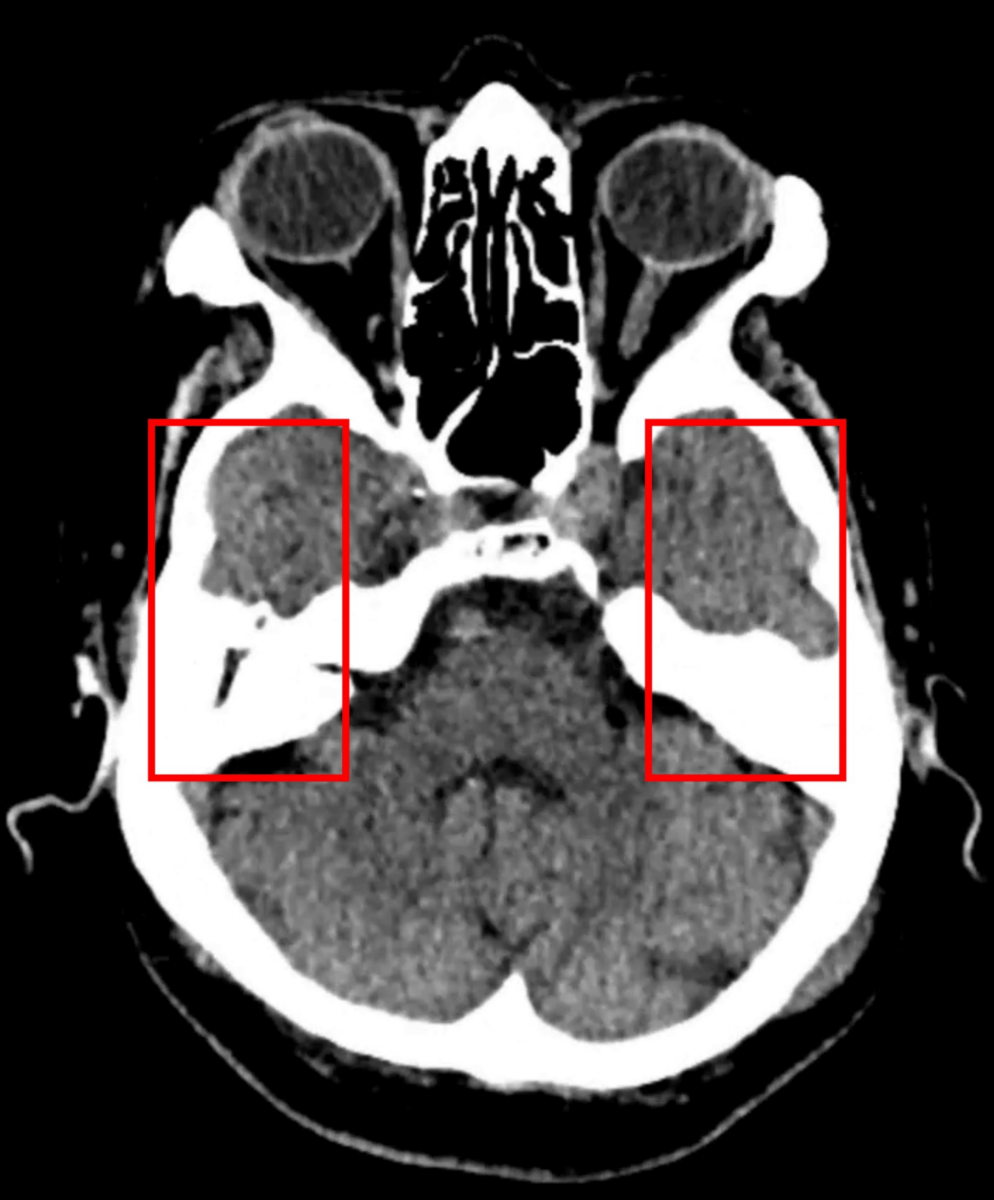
Axial CT demonstrating intact ossicular chain The ossicular chain appears intact bilaterally with preserved bony margins, making erosive infectious disease less likely.
